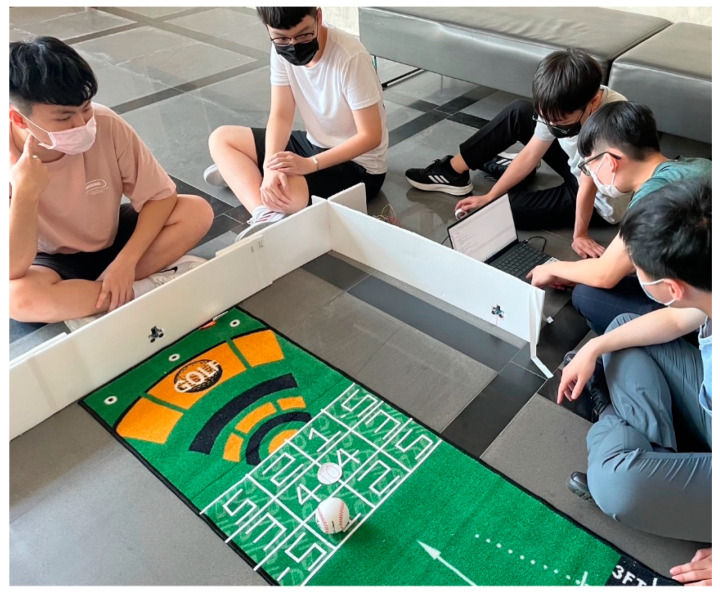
Ultrasonic sensors plate and image recognition device.
